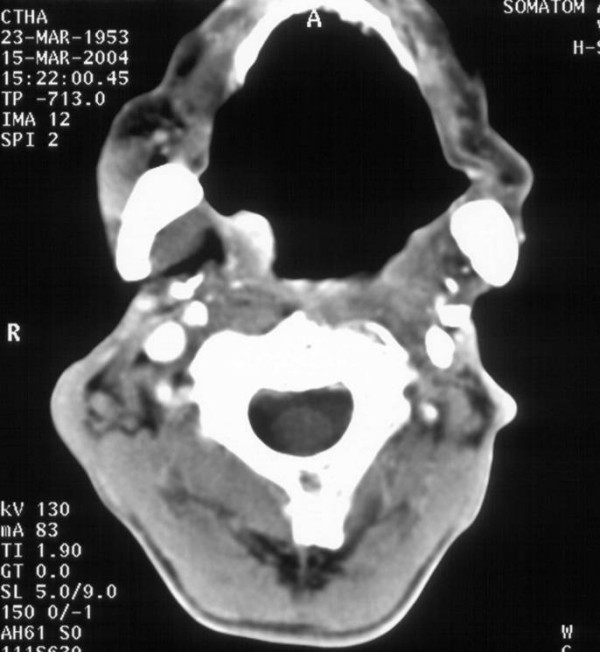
Computed tomography (CT) of the oropharynx with contrast medium. The axial projection shows a smooth bounded nodular neoformation in the right-sided tonsil's region of the oropharynx. The other layers of the tomography didn't reveal signs of enlarged cervical lymph nodes.
